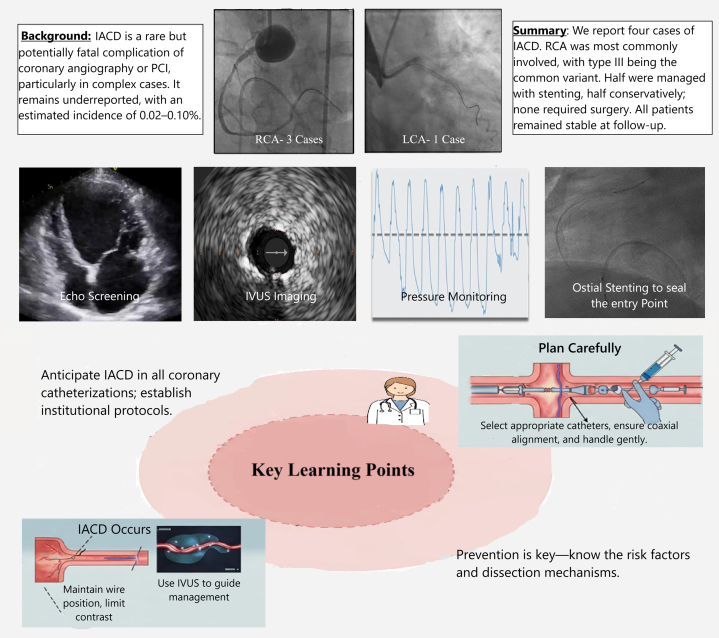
Iatrogenic Aortocoronary Dissection: A Brief Overview IACD = iatrogenic aortocoronary dissection; IVUS = intravascular ultrasound; LCA = left coronary artery; PCI = percutaneous coronary intervention; RCA = right coronary artery.
